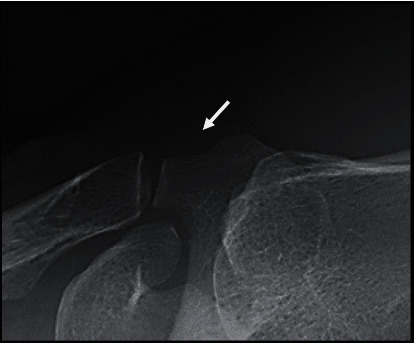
Plain radiograph with the arm in an elevated position showing the disappearance of calcification.
